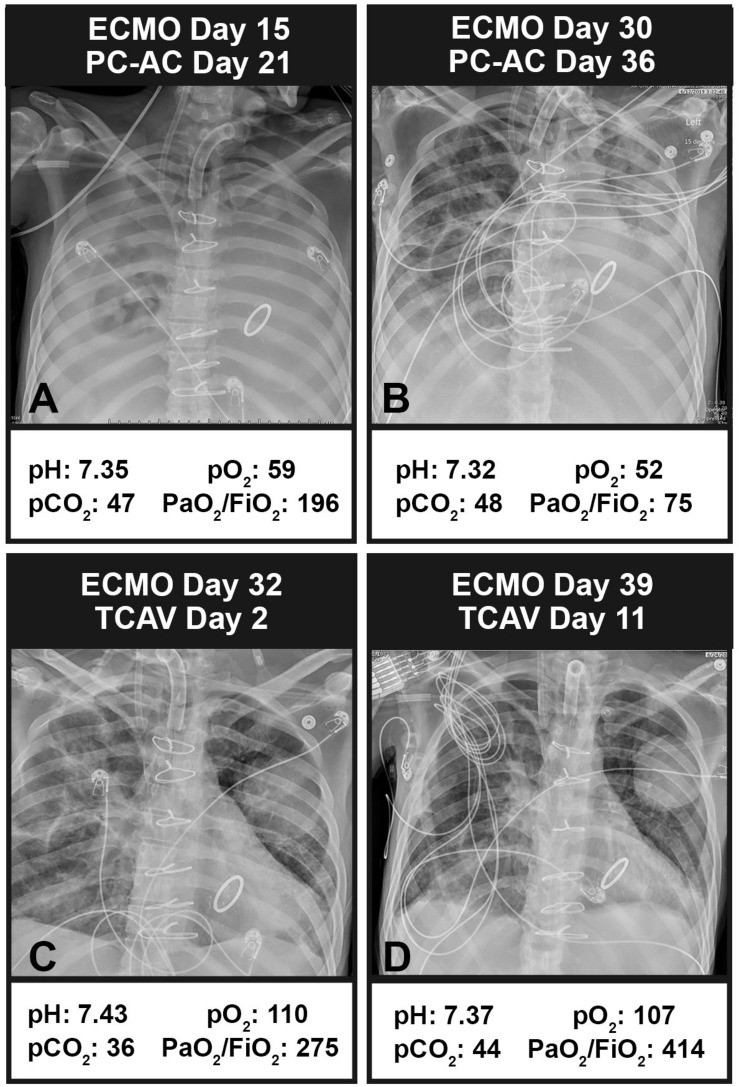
Chest X-rays (CXRs) and blood gases in a patient with respiratory failure on extracorporeal membrane oxygenation (ECMO). (A) CXR and blood gases (arterial pH, PCO_2_, and PO_2_ with the calculated PaO_2_/FiO_2_ ratio) at 15 days on ECMO and protective ARDSnet pressure-control assist-control ventilation (PC-AC). Bilateral infiltrates can be seen throughout the lung with a PaO_2_/FiO_2_ ratio of 196. (B) After 30 days on ECMO and PC-AC, the patient showed some clearing in the upper right quadrant but a worsening PaO_2_/FiO_2_ ratio of 75. (C) Two days after conversion to the TCAV method, the patient showed marked improvement in CXR and PaO_2_/FiO_2_ ratio (275). (D) After 11 days on the TCAV method, the patient showed a cleared CXR and normalized PaO_2_/FiO_2_ ratio (414). The patient came off of ECMO and was extubated. *Written informed consent obtained for the use of these images.*
